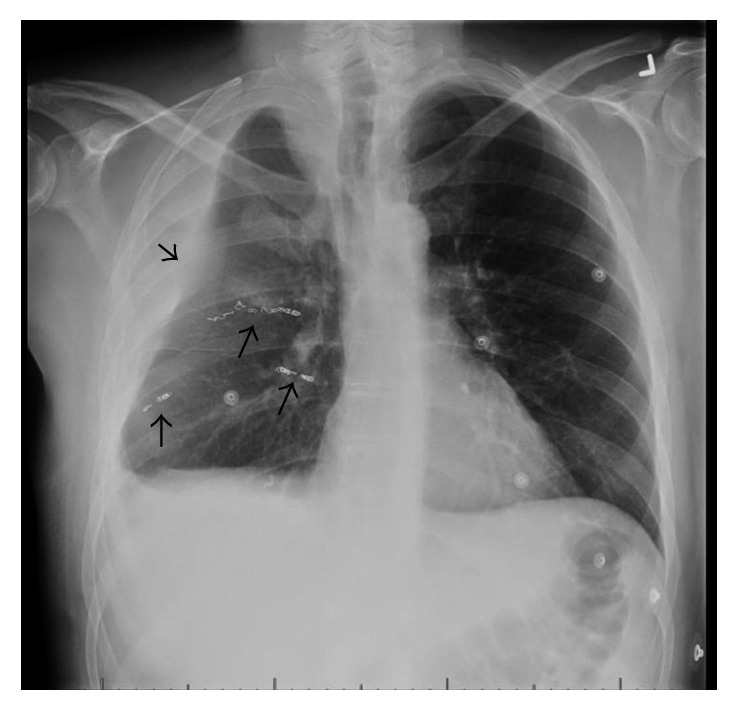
Chest X-ray of the patient on the day of discharge shows metallic coils in the intercostal arteries (arrows) and residual volume loss in the right lung (arrow head) following thoracotomy.
